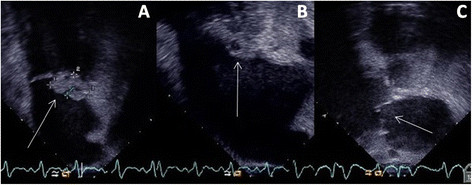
Echocardiographic imaging of the mitral valve. A: Transoesophageal echocardiogram showing the large posterior mitral valve leaflet vegetation. B: Transoesophageal echocardiogram with arrow indicating leaflet abscess. C: Transoesophageal echocardiogram post repair showing a competent mitral valve.
